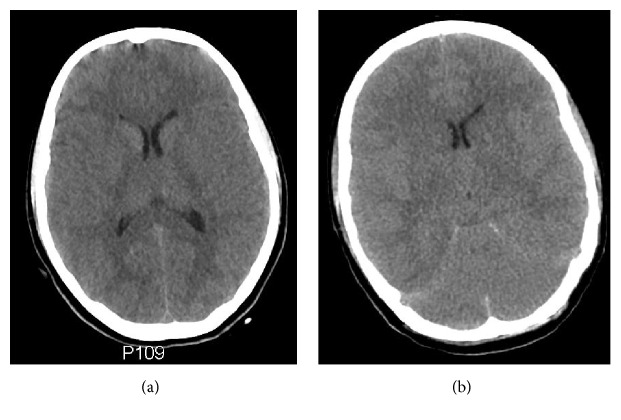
(a) Computed tomography of the brain upon arrival, showing no evidence of cerebral edema or infarction. (b) Day 3, after 7 hours of continuous renal replacement therapy: the scan now shows generalized cerebral edema with effacement of the basal cisterns and reduced grey-white matter differentiation.
